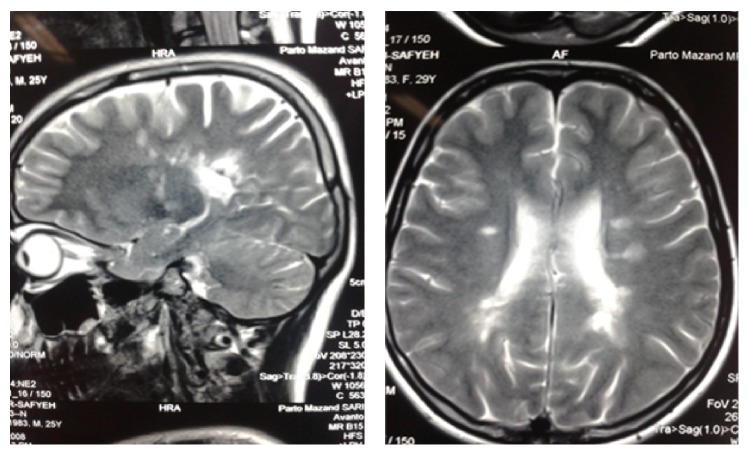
T2 MRI: periventricular plaques.
